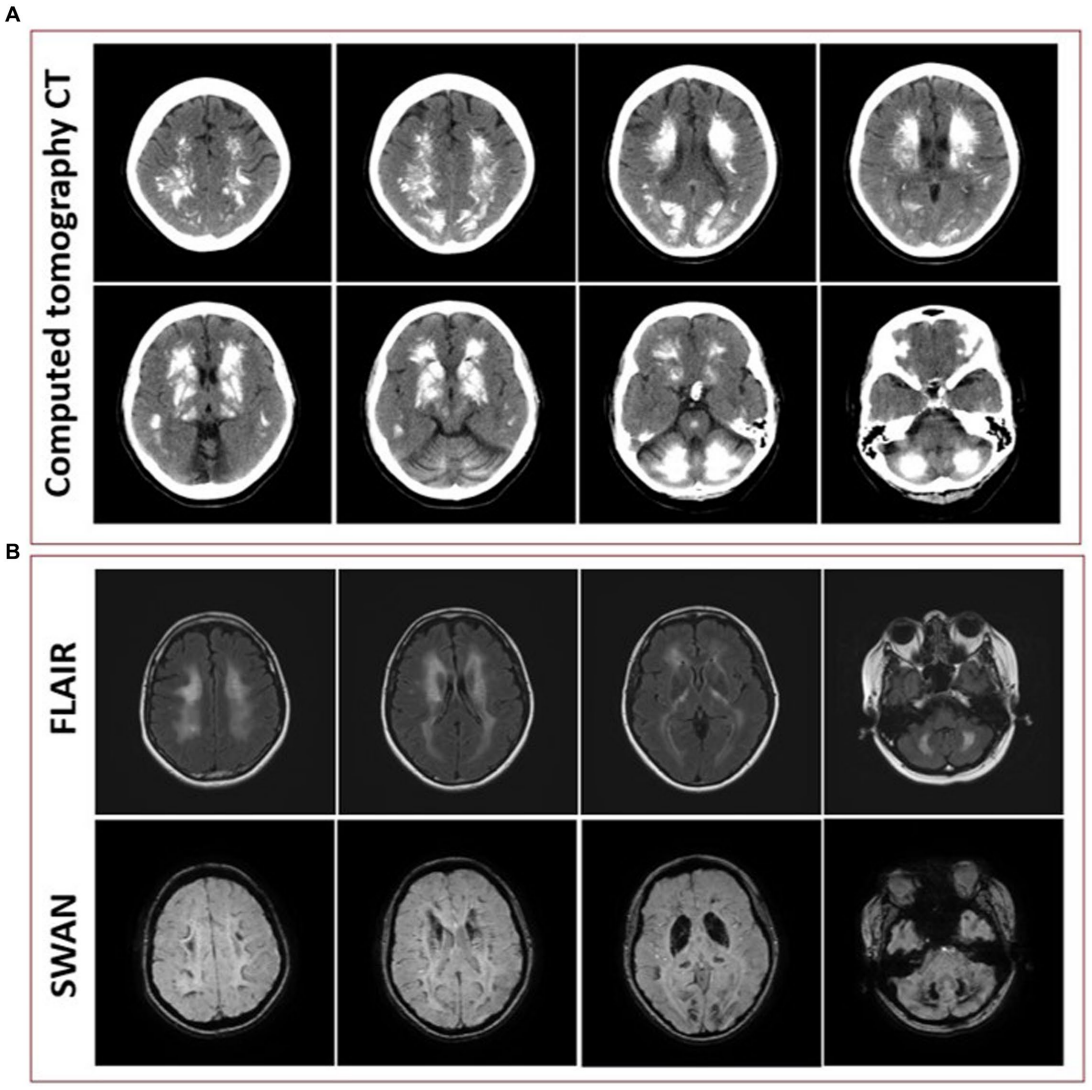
(A) Representative axial cuts from the patient’s computed tomography (CT) scan 15 months after symptom onset, showing diffuse hyperdense foci favored to represent extensive calcifications throughout the bilateral corona radiata, basal ganglia, cerebellar hemispheres, and midbrain. (B) Representative axial cuts from the patient’s magnetic resonance imaging (MRI) 15 months after symptom onset, fluid-attenuated inversion recovery (FLAIR) sequence showing high T2 signal in the bilateral cerebral, brainstem, and cerebellar deep gray nuclei and white matter and susceptibility-weighted angiography (SWAN) sequence showing extensive susceptibility effect.
